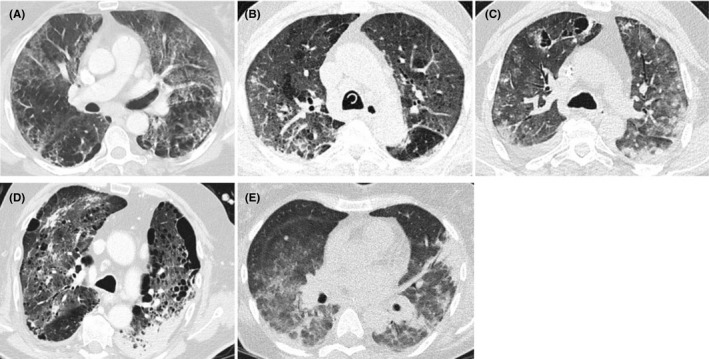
Chest CT images of patients with COVID‐19 Associated Invasive Pulmonary Aspergillosis. A, Patient #1: Combined bilateral ground‐glass opacities with crazy paving and peripheral nodular consolidations. B, Patient #2: Ground‐glass opacities with small nodular infiltrations of up to 1 cm. C, Patient #3: Bilateral ground‐glass opacities diffuse nodular infiltrates and cystic cavities and partly air crescent sign. D, Patient #4: Bullous emphysema and ground‐glass opacities, interstitial changes and consolidations with nodular infiltrates. E, Patient #5: Ground‐glass opacities, smaller areas with crazy paving pattern, central and peripheral consolidations, and smaller nodular infiltrates
